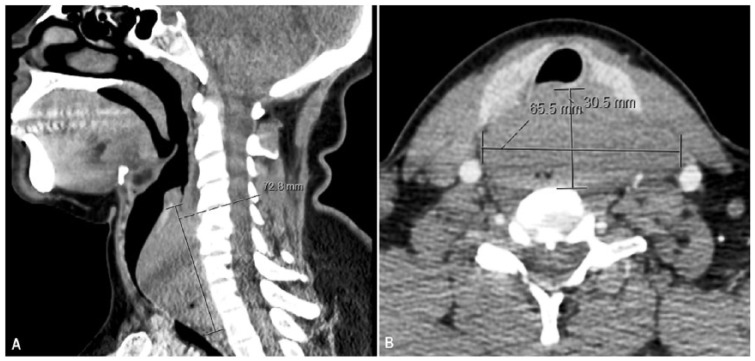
Computed tomography scan of the head and neck. (A) A 7.3 × 3.1 × 6.6-cm mass in the neck, located adjacent to the esophagus. (B) The lesion appeared to be closely apposed with the vertebral body without apparent compression.
